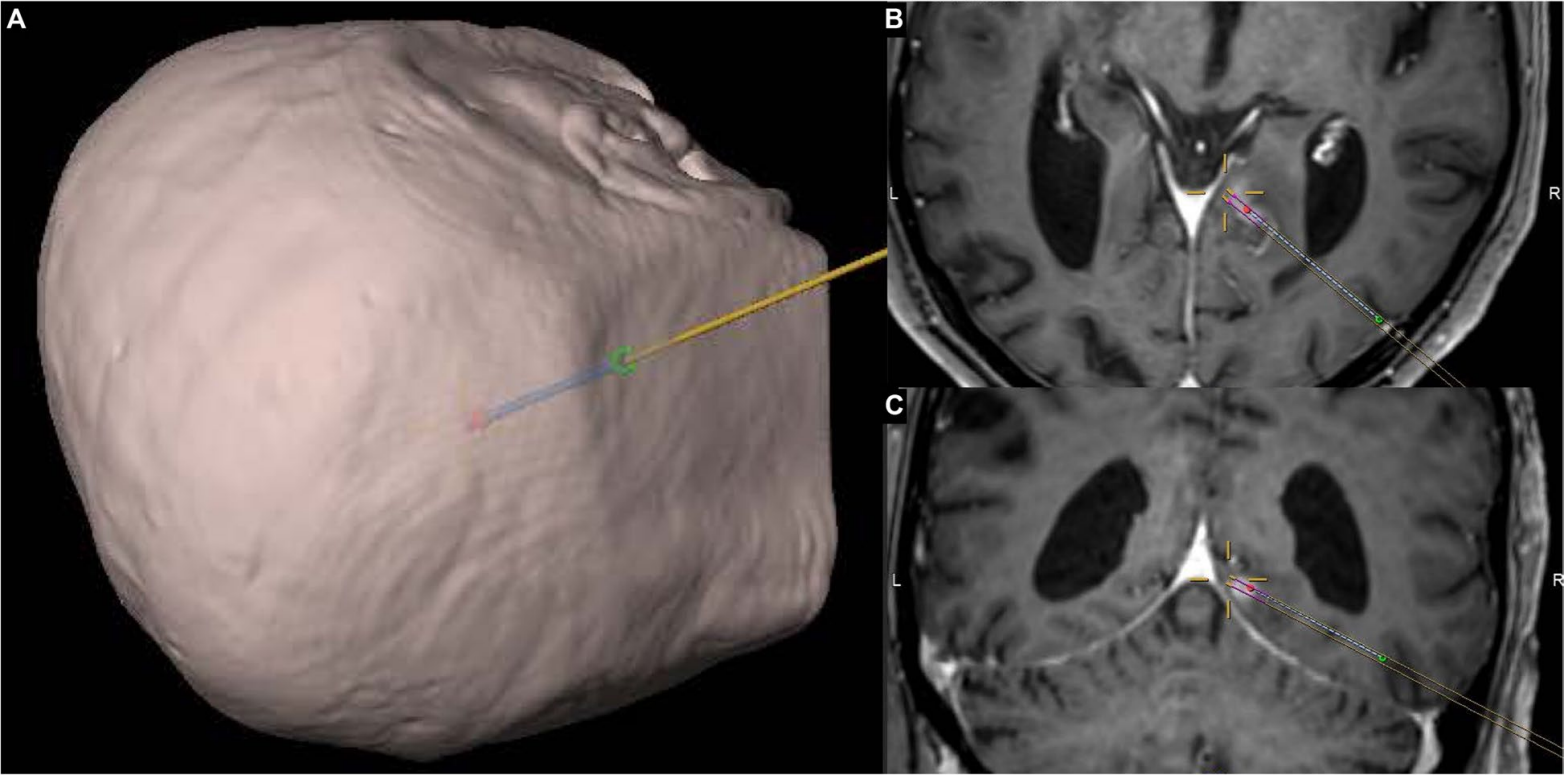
Stereotactic planning and cannula position verification in an exemplary patient. A three-dimensional image-based head reconstruction with planned biopsy trajectory from a right occipital approach. B axial and C coronal T1-weighted contrast-enhanced MRI sequences. The target is indicated by the crosshairs; mainly out of plane are the planned trajectory (parallel lines), the planned biopsy region (violet), as well as the intra-procedural CT-verified tip, intracranial trajectory and entry point of the biopsy cannula (red, dashed line and green point, respectively)
